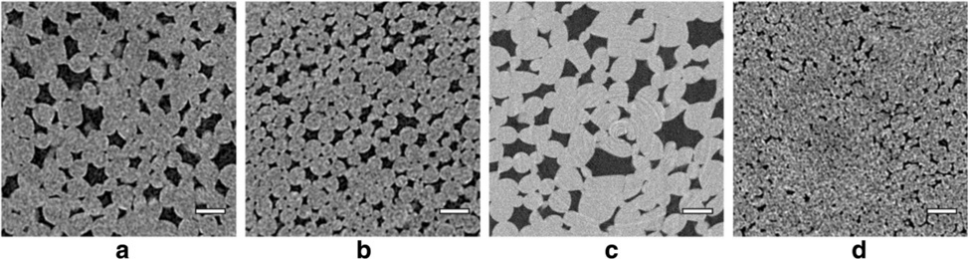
Original X-ray micro CT virtual cross-sectional images of ‘Braeburn’ (a), ‘Kanzi’ (b), ‘Jonagold’ (c) and ‘Conference’ (d) microstructure, obtained by means of a desktop CT system (a, b, d) or in a large scale synchrotron radiation CT facility (c). Cells and intercellular space are visible in all the images. Scale bar indicates 250 μm
